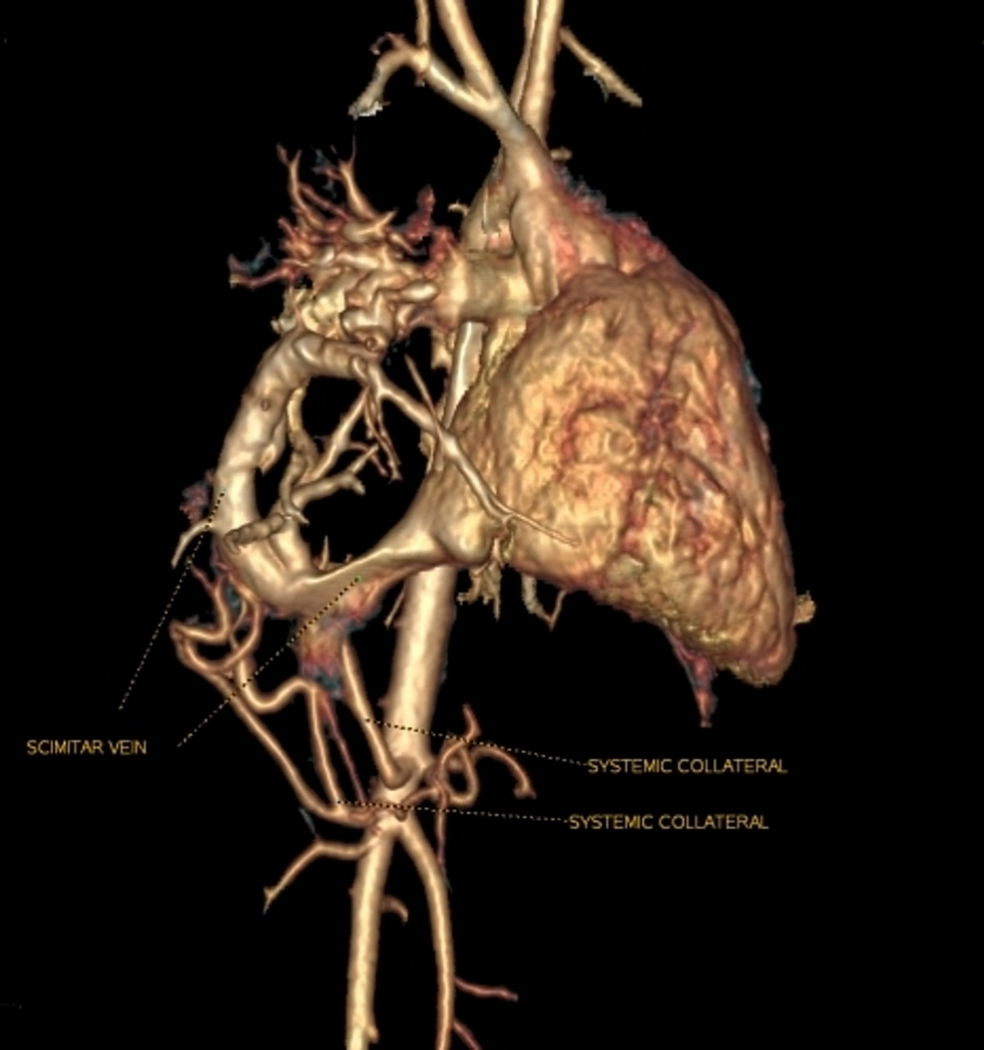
CT cardiac dynamic study volume rendering image showing scimitar veins and systemic collaterals (right lateral view)
